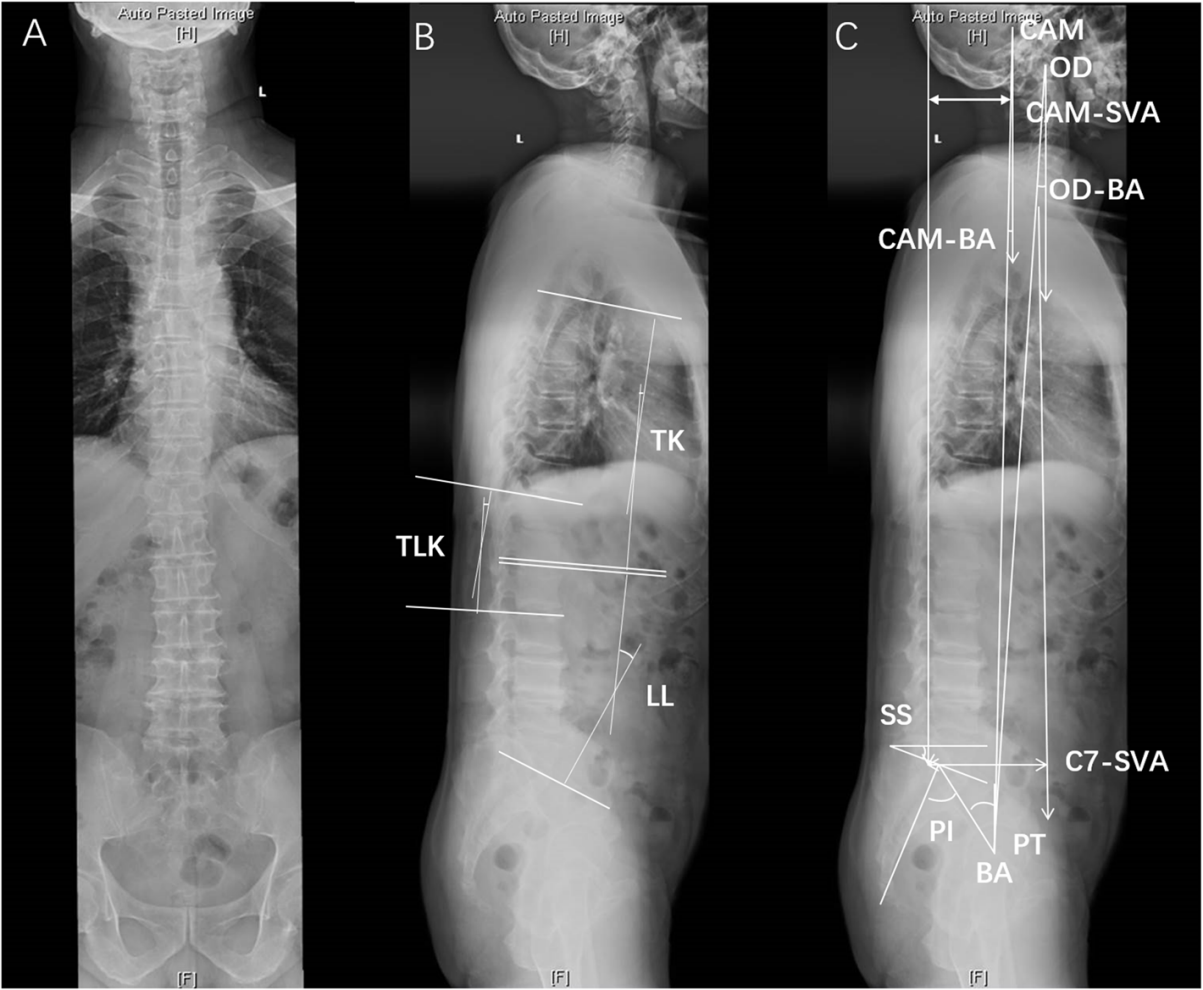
Measurements of head-spinal-pelvic parameters using the lateral full-length spine X-ray. A Anteroposterior x-ray; B and C Lateral x-ray. CAM: center of acoustic meati; SVA: sagittal vertical axis; BA: bicoxofemoral axis; TK: thoracic kyphosis; TLK: thoracolumbar kyphosis; LL: lumbar lordosis; PI: pelvic incidence; PT: pelvic tilt; SS: sacral slope
